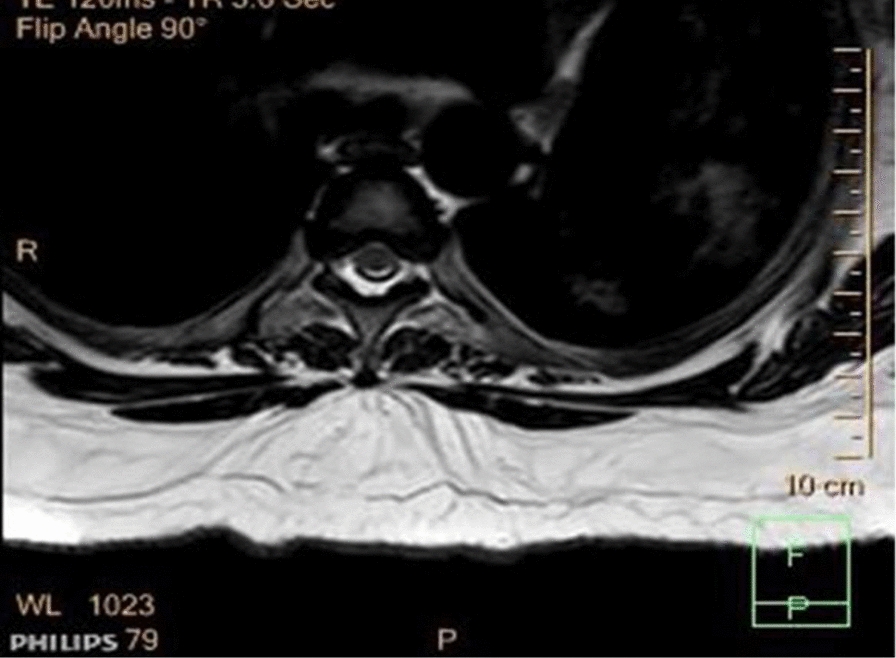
MRI of the spine. Technique T2W1, T1W1 (post-contrast), transverse view shows: STIR intensity in the intramedullary segment of the thoracic spinal cord and loss of volume of the spine more of the posterolateral aspect
